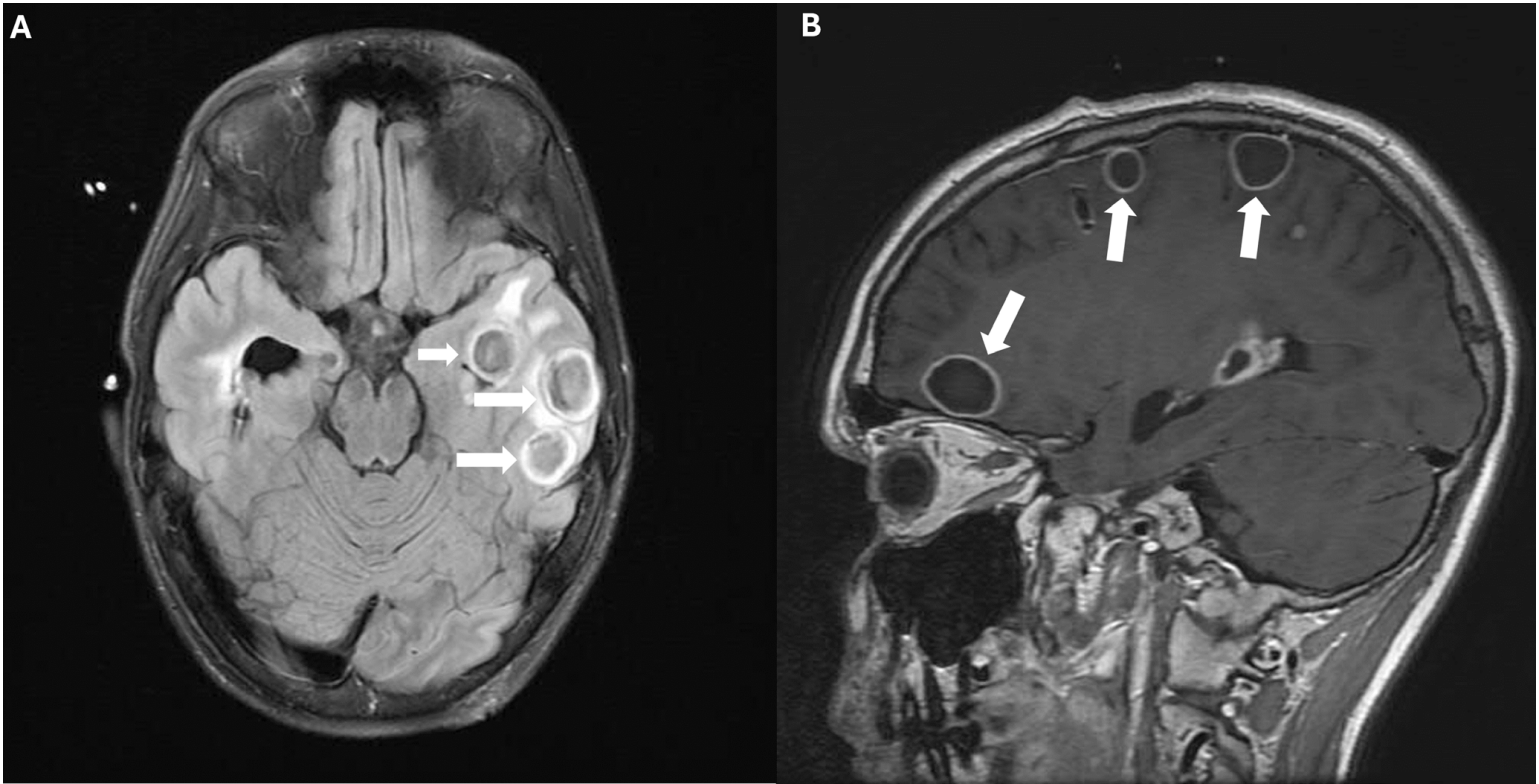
A and B Axial MRI images demonstrating resolving brain abscesses. White arrows highlight the reduced size of abscesses
